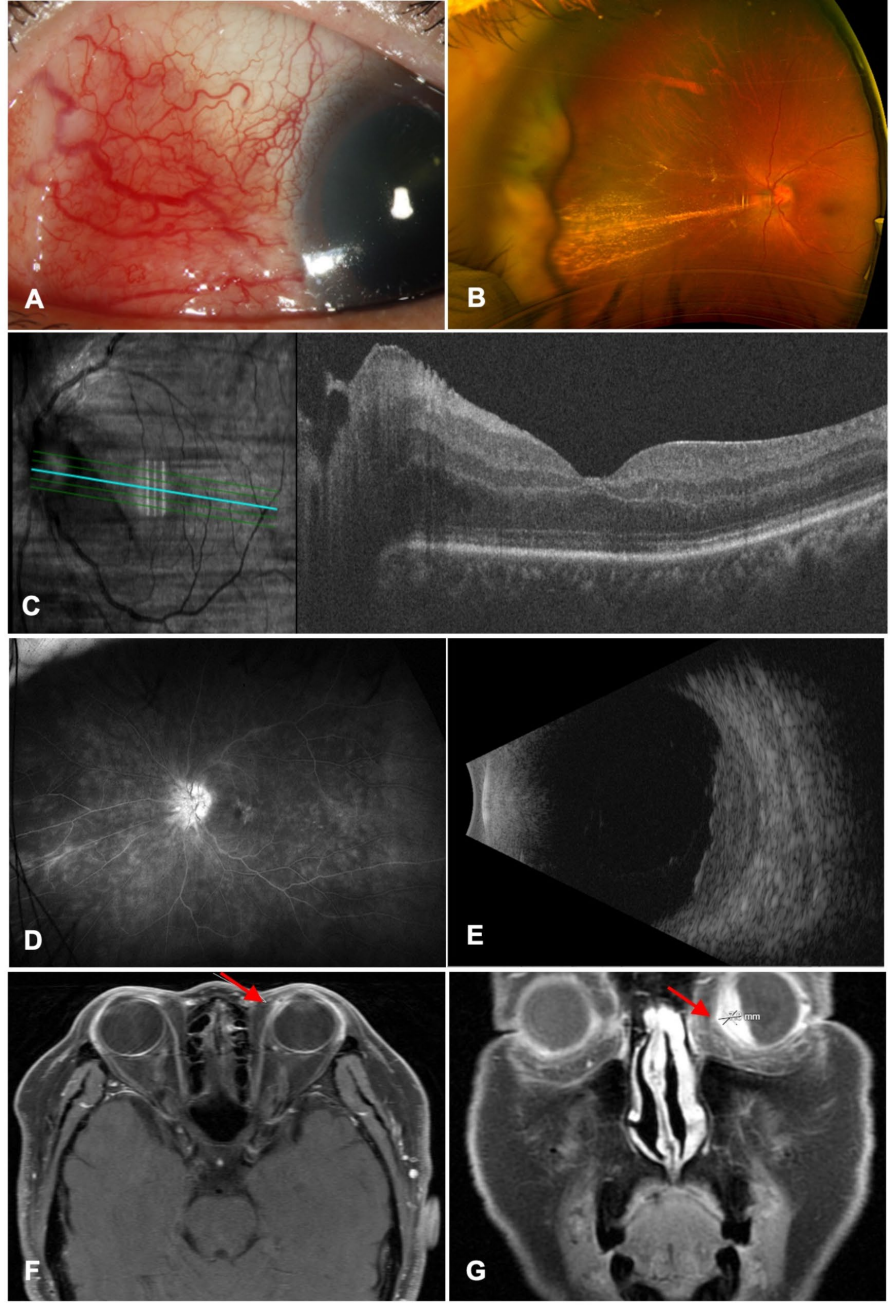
Selected images demonstrating finding of scleritis and ciliary body mass at presentation (left eye). A) Slit-lamp photograph showing 3 + scleral injection consistent with active anterior scleritis in nasal quadrant; B)  *Fundus photograph showing perivascular exudates and choroidal mass in the nasal retina*; C) Optical coherence topography showing macular thickening nasal to the fovea; D) Fluorescein angiography showing diffuse leakage of the optic disc and peripheral retinal vessels; E) Ultrasonography showing choroidal thickening with mild serous choroidal detachment; F) Magnetic resonance imaging showing enhancing lesion (arrow) in the left anterior nasal cavity with extra-nasal extension; G) Magnetic resonance imaging showing enhancing lesion along the medial ciliary body (arrow) in the left eye with extension to vitreous cavity
